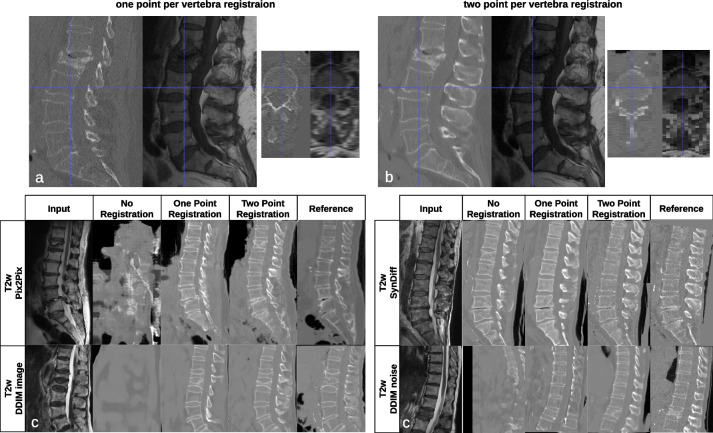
Comparison of one and two registration points per vertebra *versus* real data. a We registered with a single point in the center of the vertebral body. The vertebral body could rotate along the spine axis. This caused the posterior vertebra structures to be misaligned. b When we registered the images with an additional point on the spinous process, we avoided this rotation around the spine itself. The blue dashed lines are for locating the relation between axial and sagittal slices. c Translation with networks trained on registrations with 0, 1, or 2 points per vertebra. Images are from the in-house T2-weighted test dataset. Posterior structures are only reconstructed correctly with 2-point registration. *DDIM* Denoising diffusion implicit model
